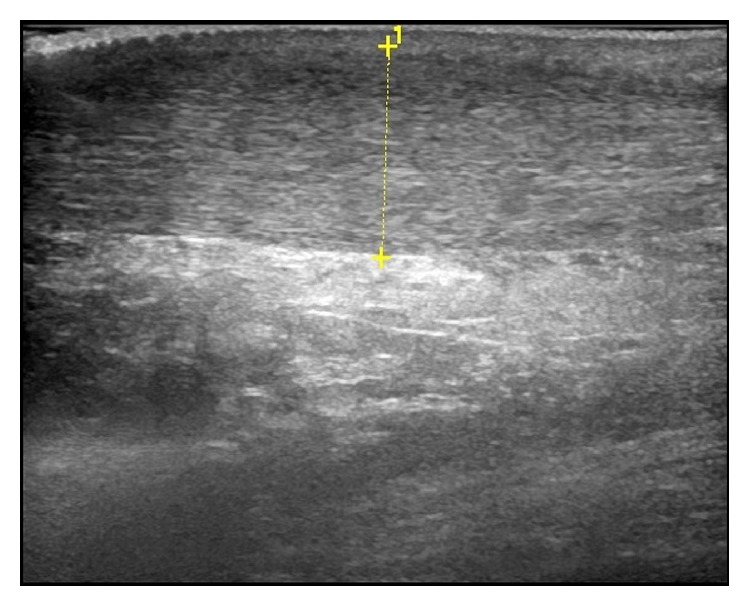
Pretreatment ultrasound image of the Achilles tendon.
